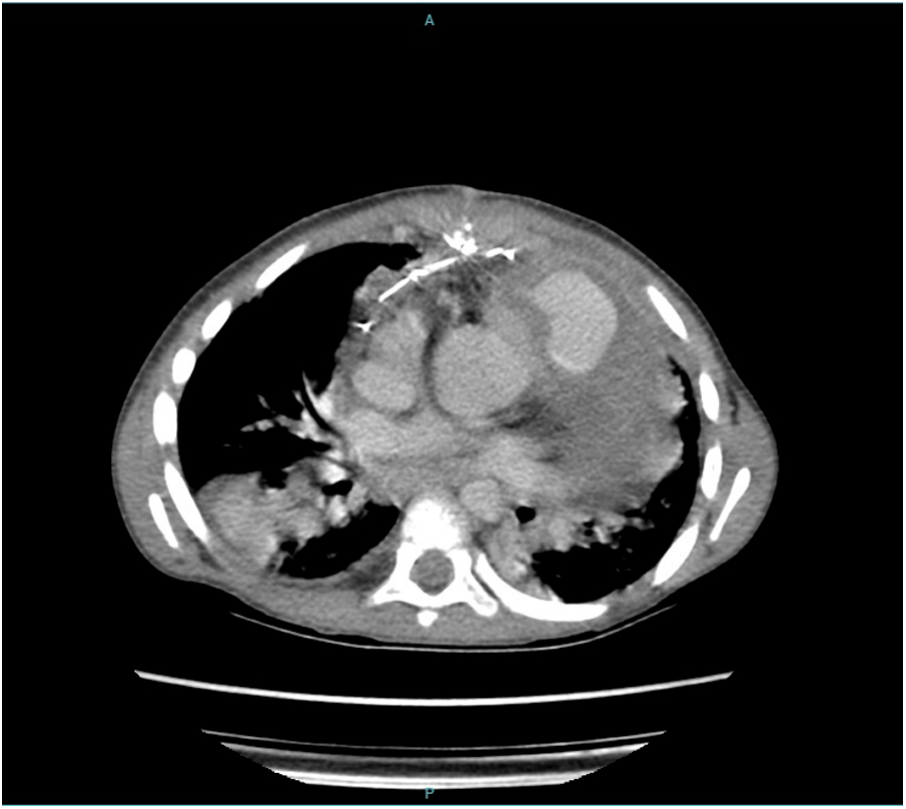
CT scan showing images compatible with pulmonary necrosis in the upper segment of the right lower pulmonary lobe.
